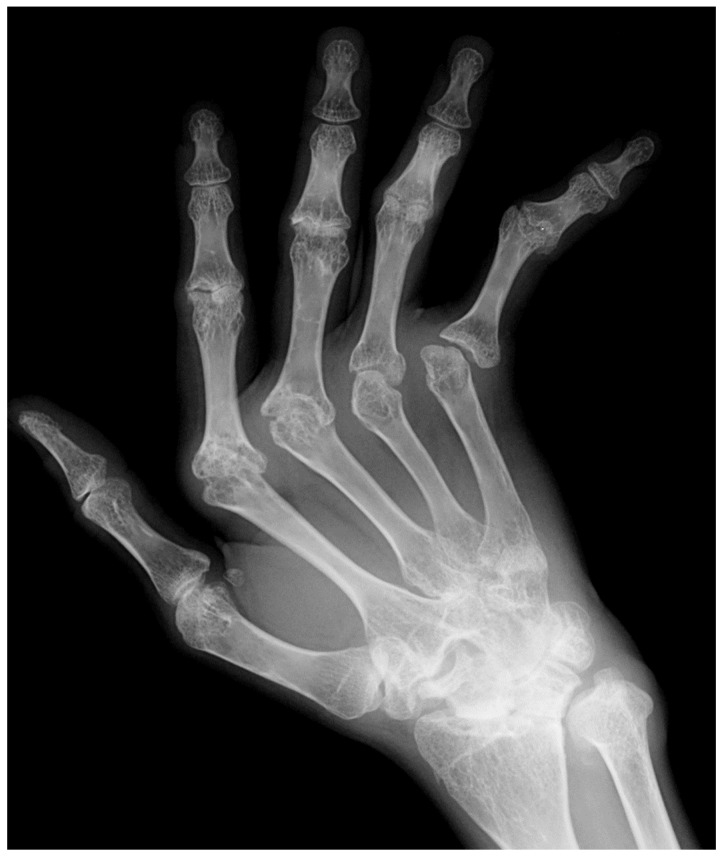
60-year-old female with long-standing rheumatoid arthritis. Hand radiographs shows erosive changes in the PIP and MCP joints as well as the wrist. Ulnar subluxation of the fingers at MCP joints is also present. Note the distal interphalangeal joints are spared.
